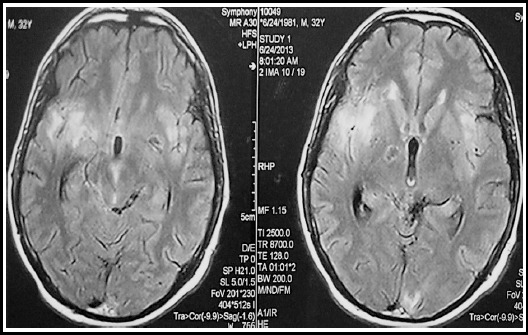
Axial FLAIR-weighted MRI images of 32 years old man shows multiple high signal intensity (SI) lesions in bilateral temporal lobes and the basal ganglia representing vasculitis and small infarctions.
